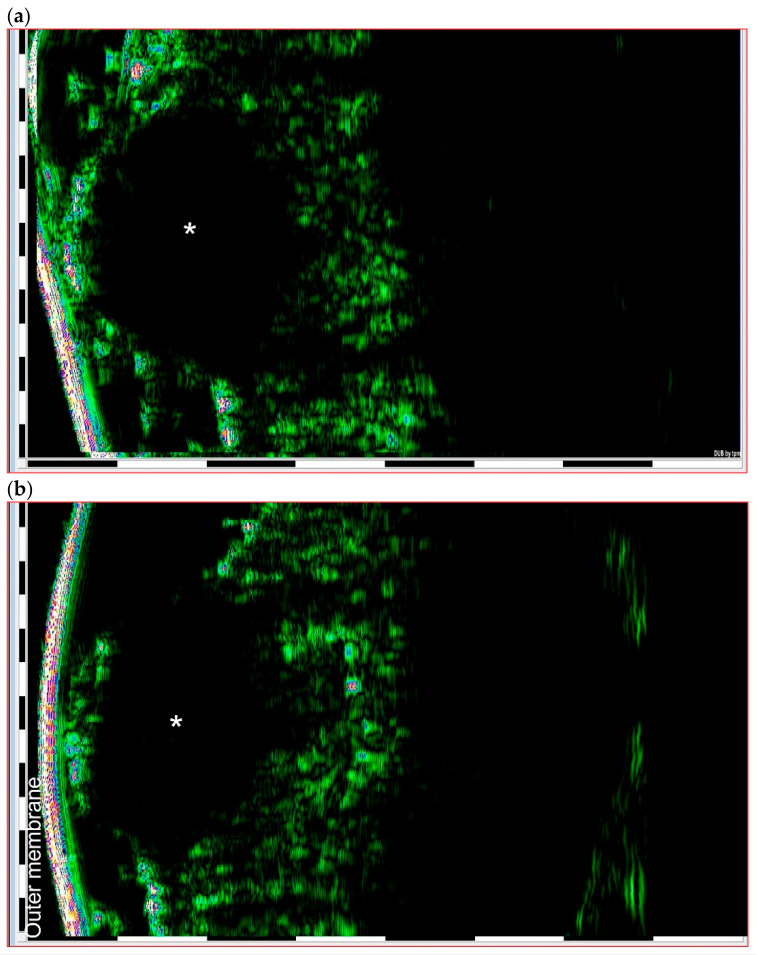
HFUS BCC images of a nodular BCC (round shape with well-defined margins) (a) before surgical excision (in vivo HFUS), and (b) the same tumor after excision (ex vivo HFUS). The star corresponds to the center of the tumor; the outer membrane is the film placed between the ultrasound head and the skin for protection.
